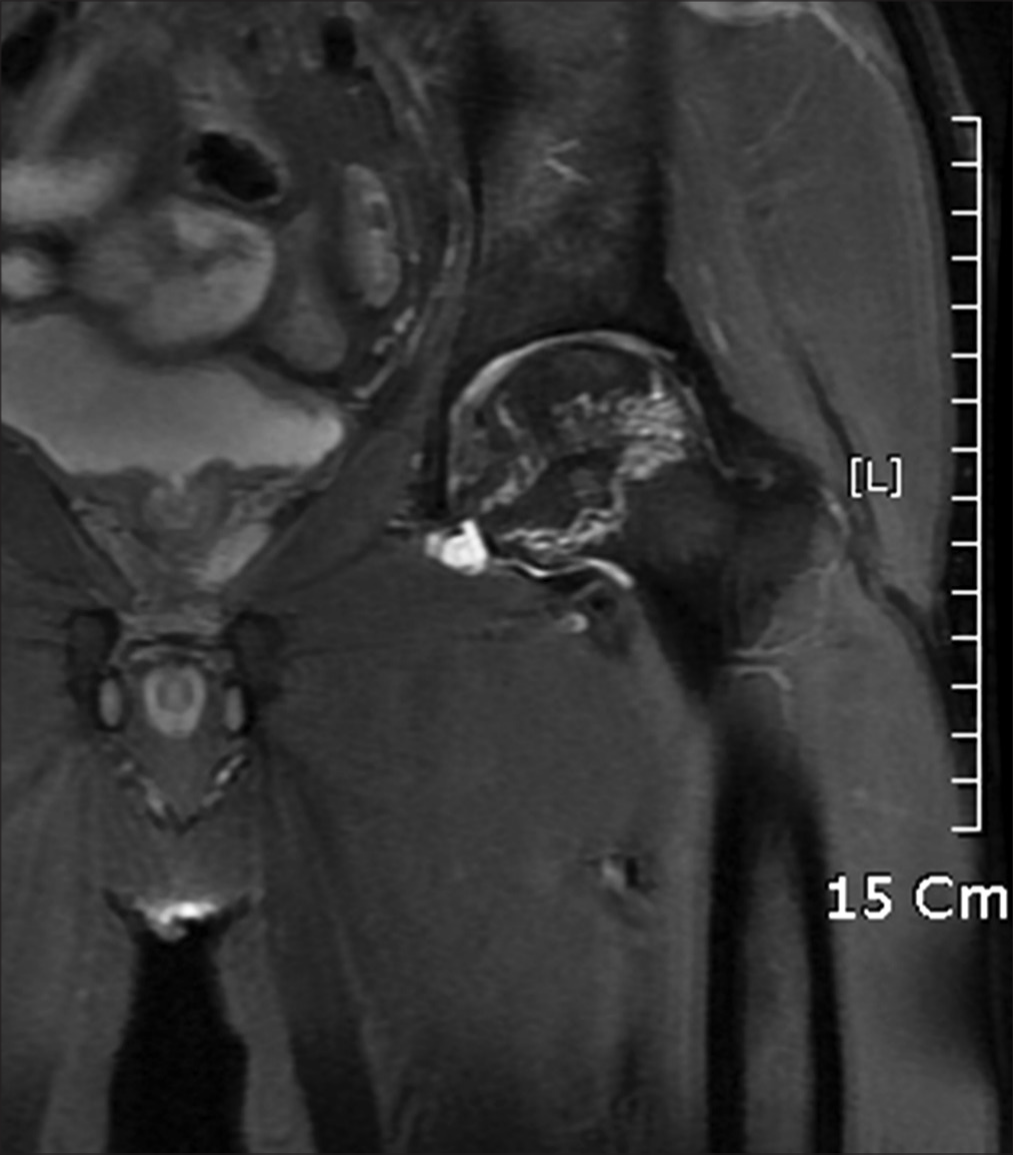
MRI scan showing large osteonecrotic lesion involving more than 80% geographical area of articular surface of the head of femur with MR crescent sign
